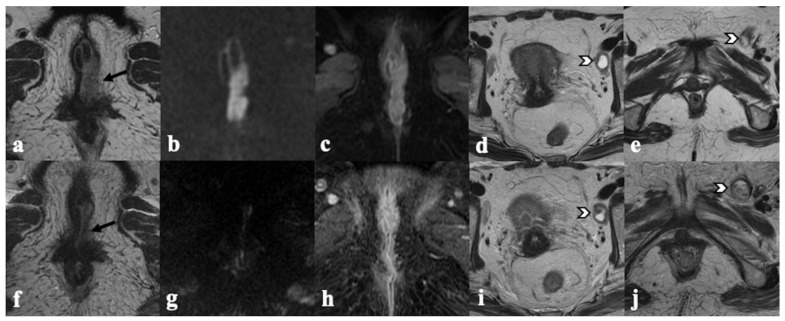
Partial response after CRT. A 73-year-old patient with locally advanced vulvar cancer (FIGO stage IVA) presenting with an intermediate T2 signal intensity mass involving left labia minor and major ((a)—black arrow), with hyperintensity in DWI (b) and enhancement in T1-WI post-contrast (c) and ulcerated pelvic ((d)—white arrowhead) and left inguinal ((e)—white arrowhead) lymph nodes. MRI after CRT (bottom row) shows significant lesion size reduction in both T2W ((f)—black arrow), DWI (g) and post-contrast T1W images (h), but dimensional increase in regional lymph nodes ((i,j)—white arrowheads), consistent with partial response.
